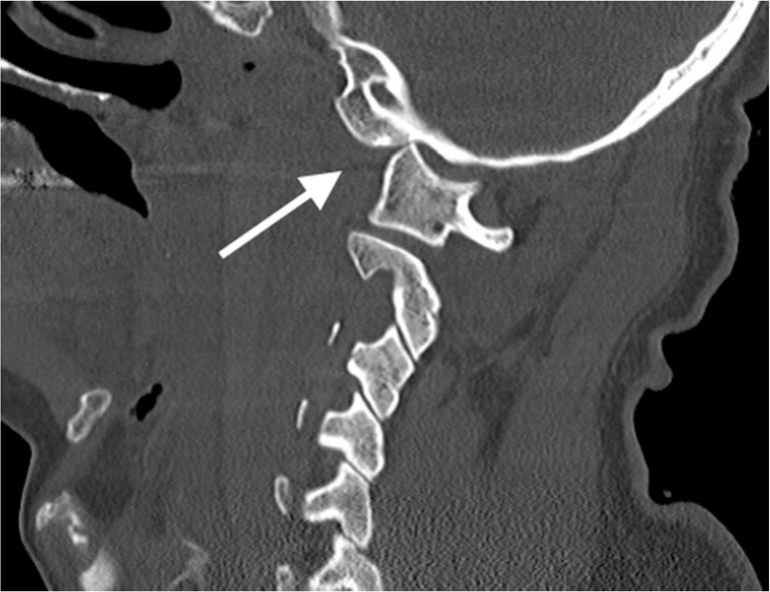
Anterior atlanto-occipital dislocation (type I dislocation): sagittal (right) reformatted CT image of craniocervical junction of a post-mortem CT study of a victim of young male patient who sustained high--force impact to the cranium as a result of an industrial accident with resultant bilateral atlanto-occipital dislocation (right joint dislocation indicated by *arrow*). The victim died at the scene: such craniocervical trauma carries a very high mortality and, as a result, ante-mortem imaging of such injuries is uncommon
